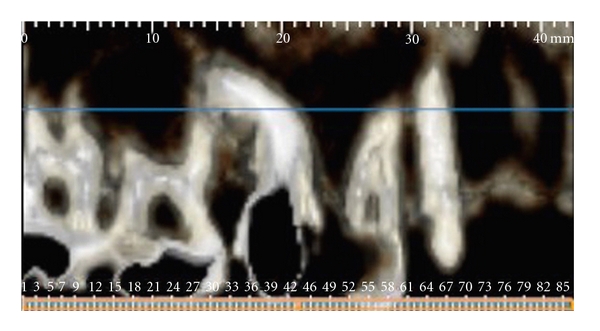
Cone-beam computerized tomography image showing close association between the roots of the maxillary second premolar and first molar.
